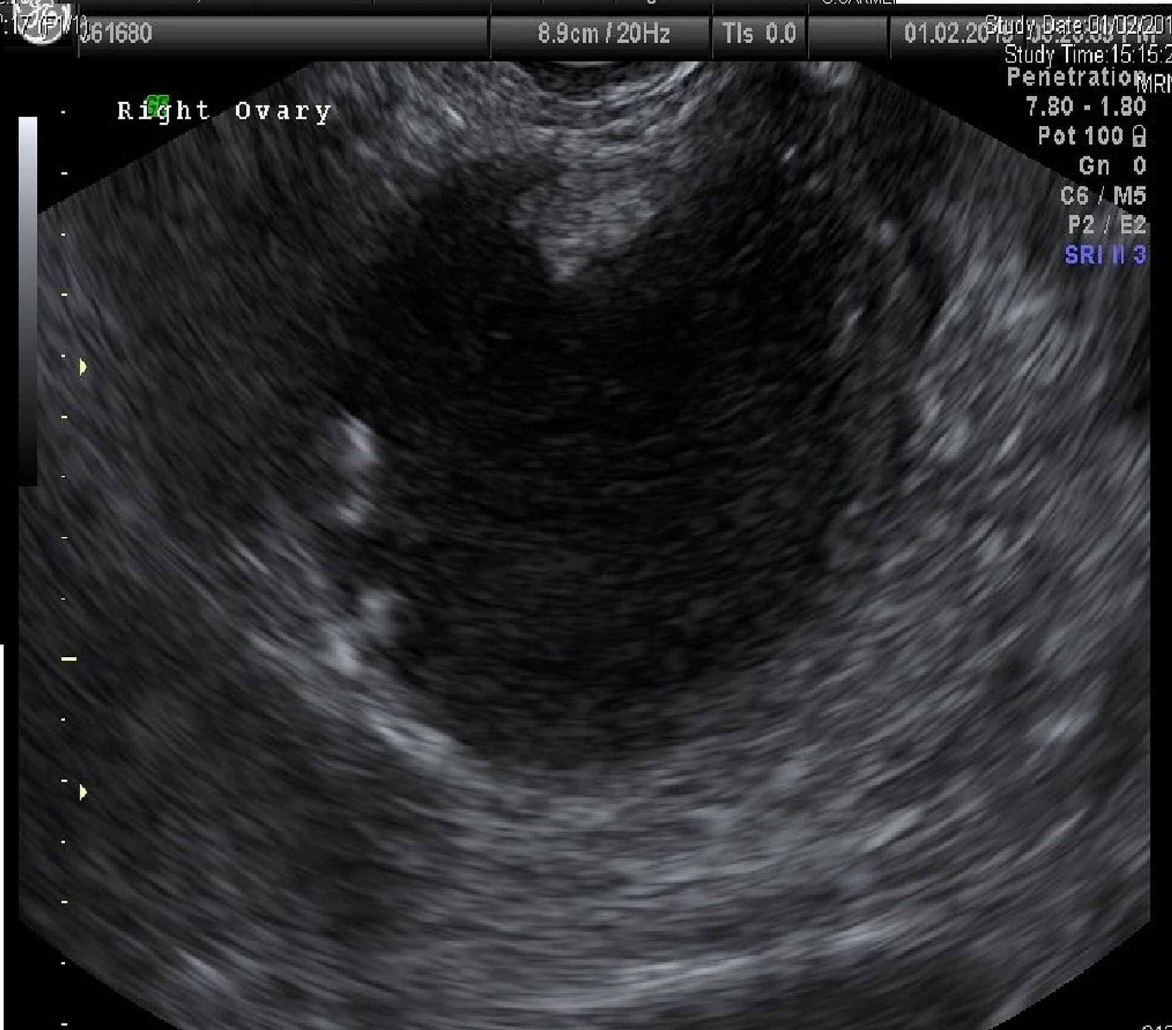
Transvaginal ultrasound: Adnexal cyst with papillary formation
